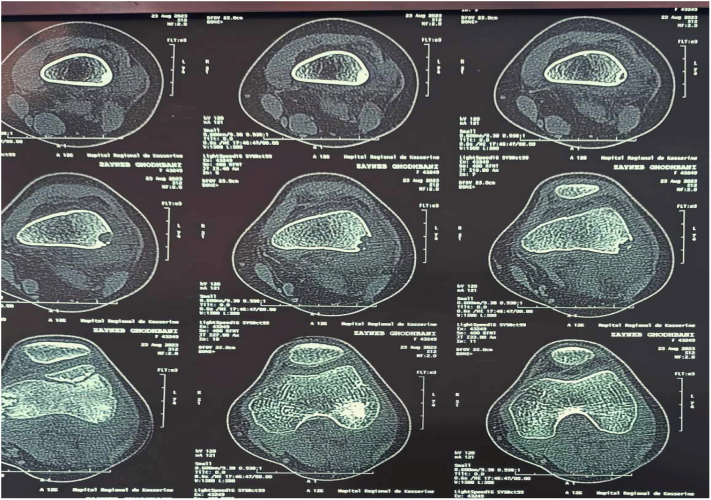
CT scan: osteolytic lesion with clear boundaries with postero-external cortical break-in.
